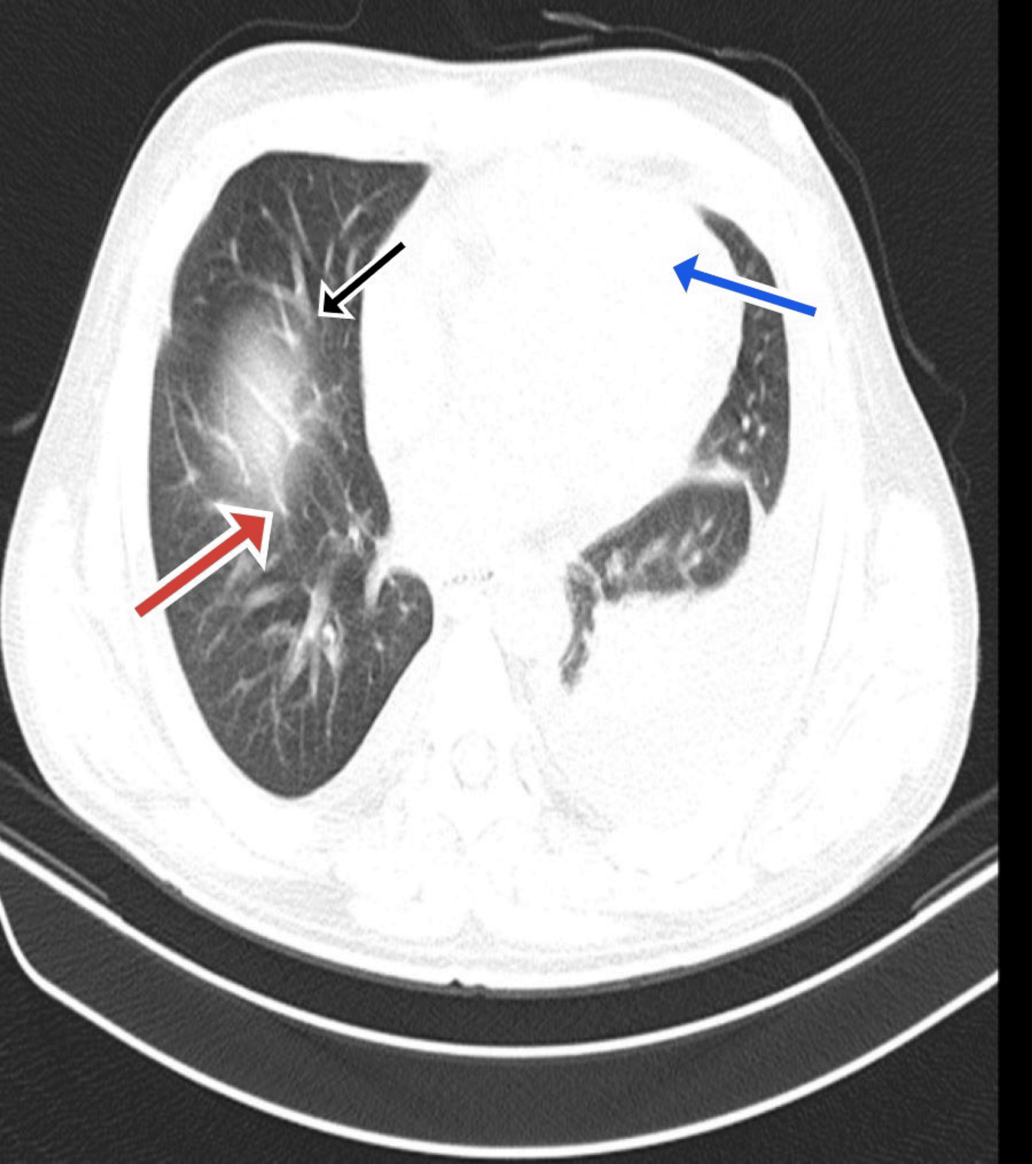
Plain CT chest showing ground-glass opacities (red arrow), pleural effusion (blue arrow), and interlobular septum thickening (black arrow).
